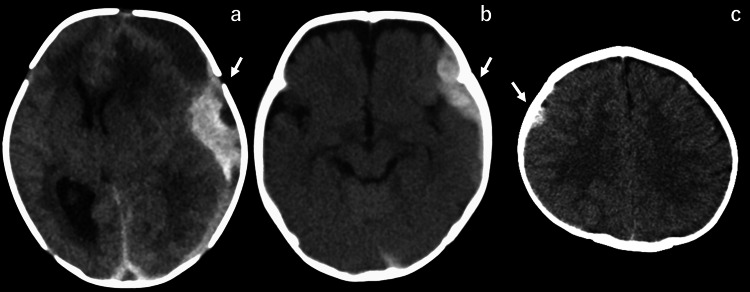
CT findings of Cases 3, 4 and 5 CT images from (a) Case 3, (b) Case 4, and (c) Case 5 show SDH in the left parietal lobe, left temporal lobe, and right frontal lobe (arrows), respectively.
